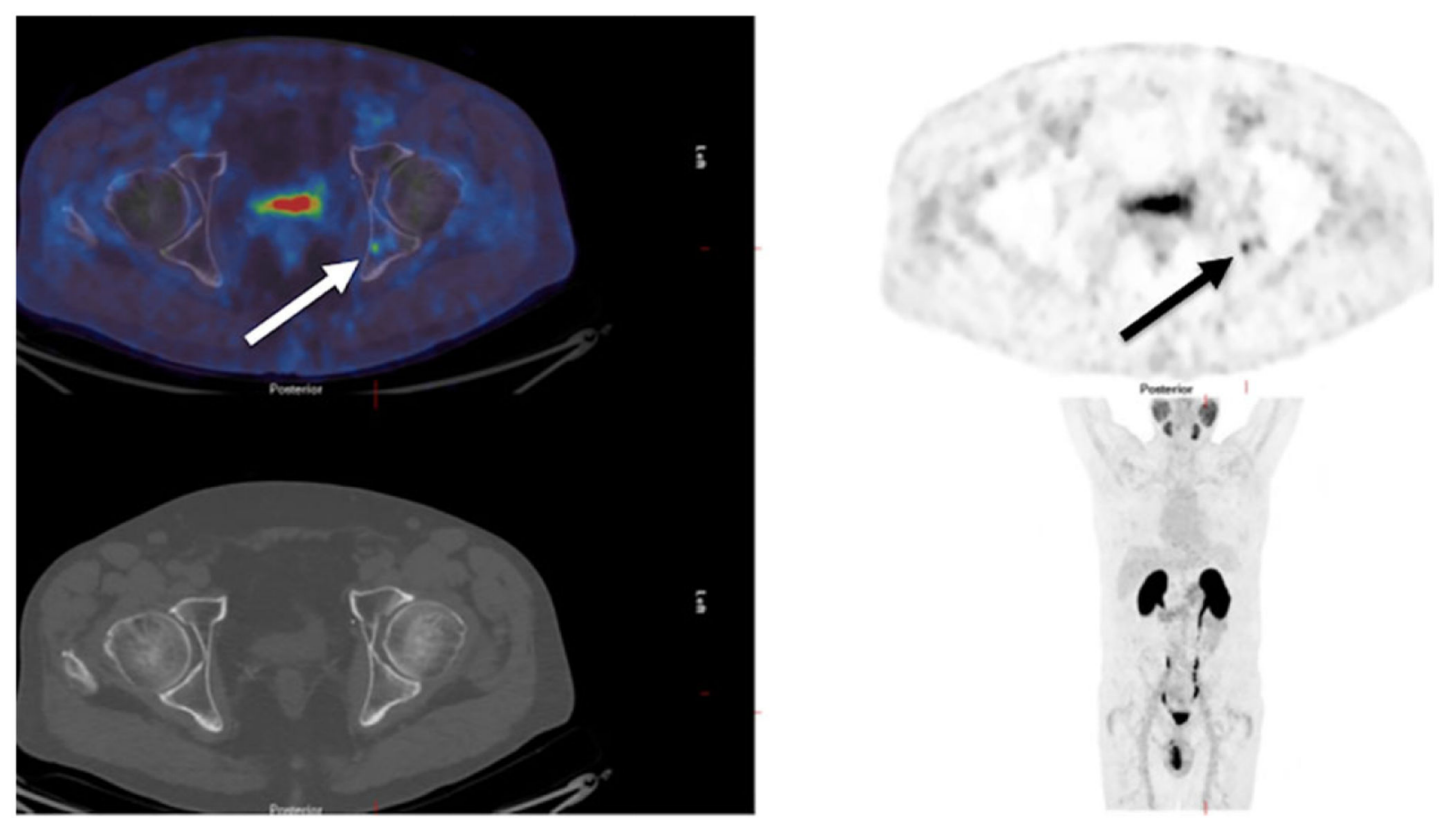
A man with biochemical recurrence of prostate cancer. A 68Ga-prostate-specific membrane antigen positron emission tomography/computed tomography (PET/CT) scan shows a small metastasis in the left posterior acetabulum (arrows), invisible on the CT component of the study (bottom left).
